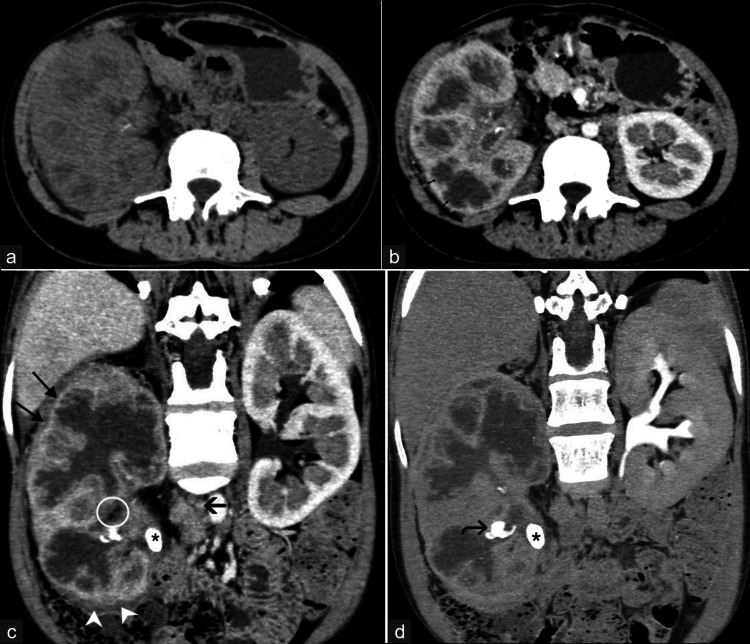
Xanthogranulomatous pyelonephritis demonstrating classic "bear paw" sign. A 32-year-old female patient complaining of right-sided abdominal pain was diagnosed with xanthogranulomatous pyelonephritis. (a) Non-contrast axial CT image shows an enlarged right kidney with edematous cortex and hydronephrosis. (b) Nephrographic phase axial CT urogram image reveals minimally enhancing heterogeneous, thinned-out right renal parenchyma (double-headed arrows) and grossly dilated pelvicalyceal system with "bear paw" sign. (c) Nephrographic phase coronal reformatted image shows moderate perinephric fat stranding (long black arrows), the thickening of renal fascia, minimal perinephric fluid (arrowhead), and a few enlarged retroperitoneal lymph nodes (short black arrow). Areas with few fat density foci (circle) are seen in the renal parenchyma. An obstructive calculus (black asterisk) is seen in the pelviureteric junction. (d) Coronal reformatted image of a four-hour-delayed phase shows no contrast opacification in the collecting system and ureter on the right side, indicating reduced function of the right kidney. A few renal calculi (black curved arrow) are also seen. These images are from the hospital and have not been published elsewhere. CT: computed tomography
